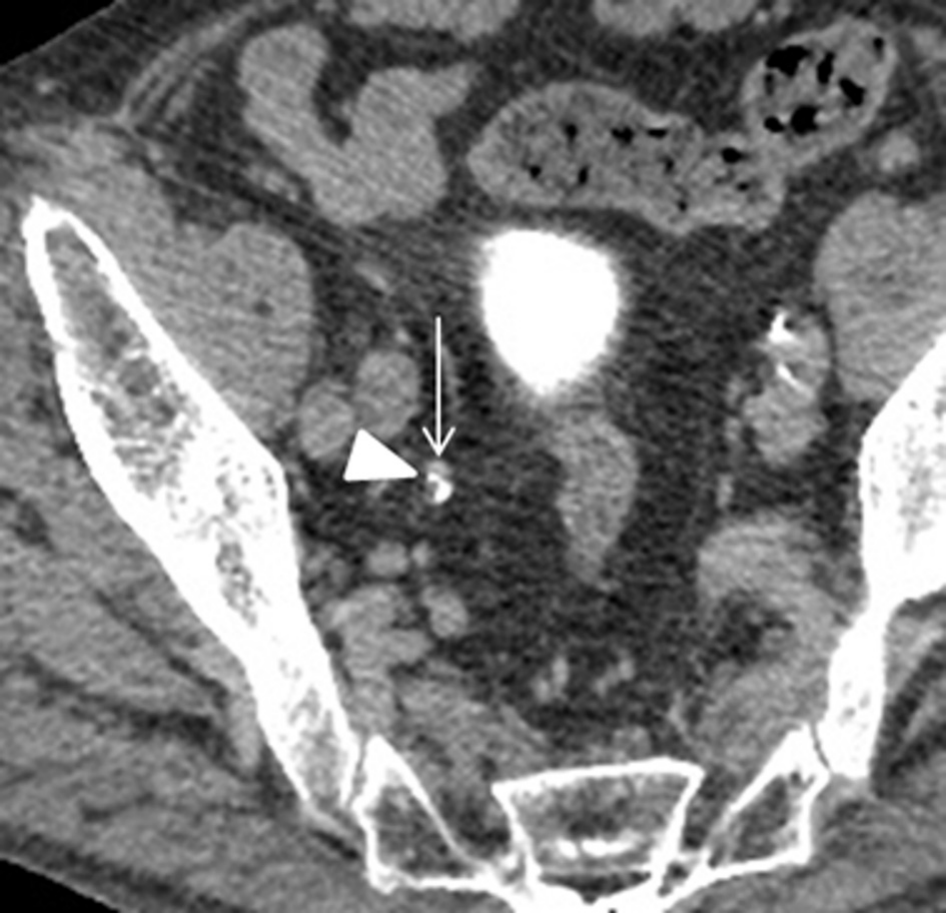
CT urography (excretory phase) During the excretory phase, a filling defect (arrow) of a few millimetres is visible in the right ureter distal to the lesion shown in Figure 1. A small ureteral cancer was suspected. A tiny outpouching of contrast medium (arrow head) possibly representing a diverticulum is visible around the tumour.
